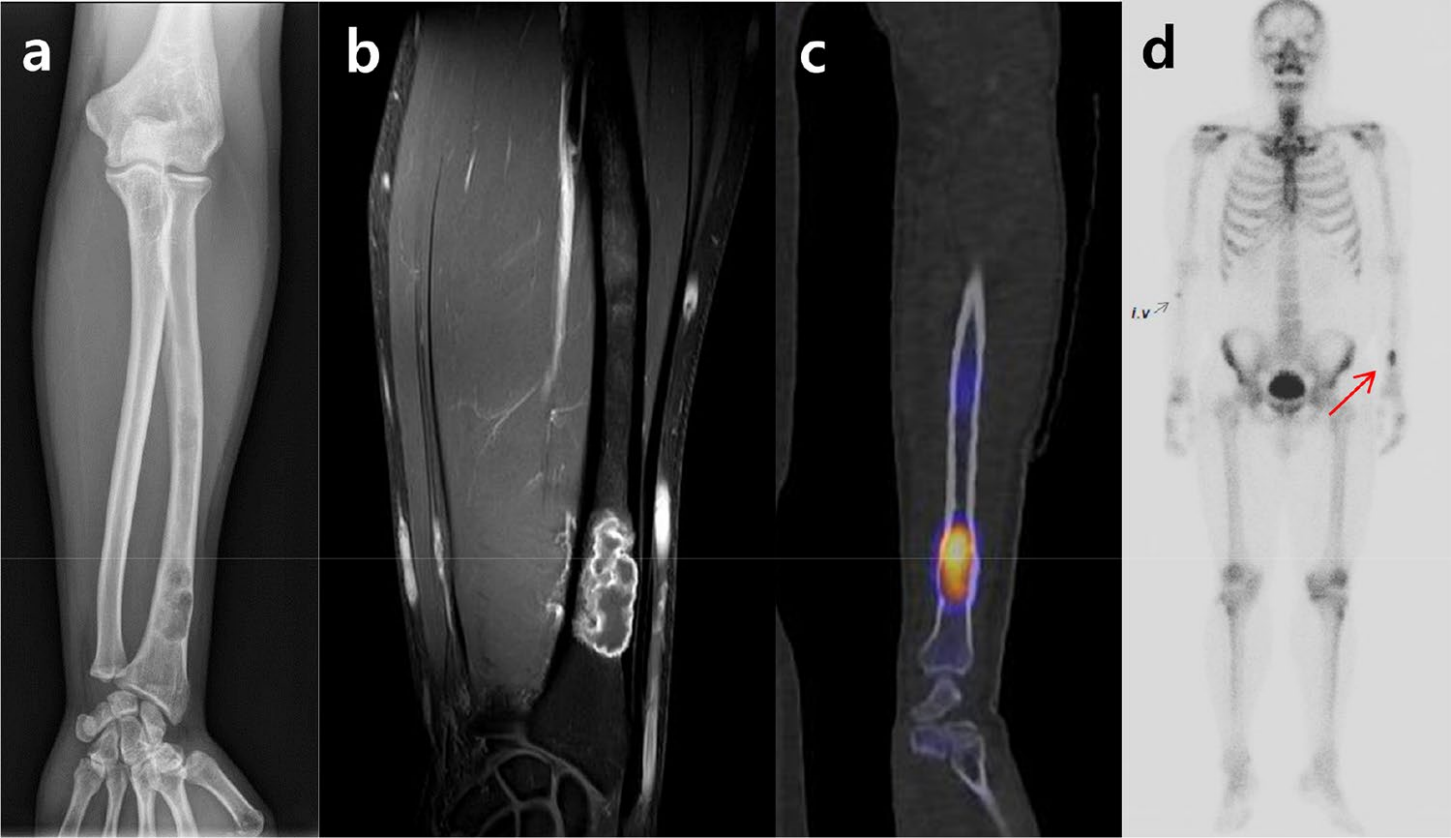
A 35-year-old male patient who was diagnosed grade I chondrosarcoma. (a) A geographic osteolytic lesion with cortical erosion was incidentally detected at metaphysis of left distal radius. (b) On fat-suppressed contrast-enhanced T1-weighted images, a 3.3 cm sized lobulated mass with rim and septal enhancement and endosteal scalloping involving more than two third of cortical thickness is shown. (c) On SPECT/CT images, the SUVmax of this lesion was calculated to be 22.9. (d) Whole body bone scan image shows focal radionuclide uptake equal to the anterior iliac crest in left distal radius (arrow).
